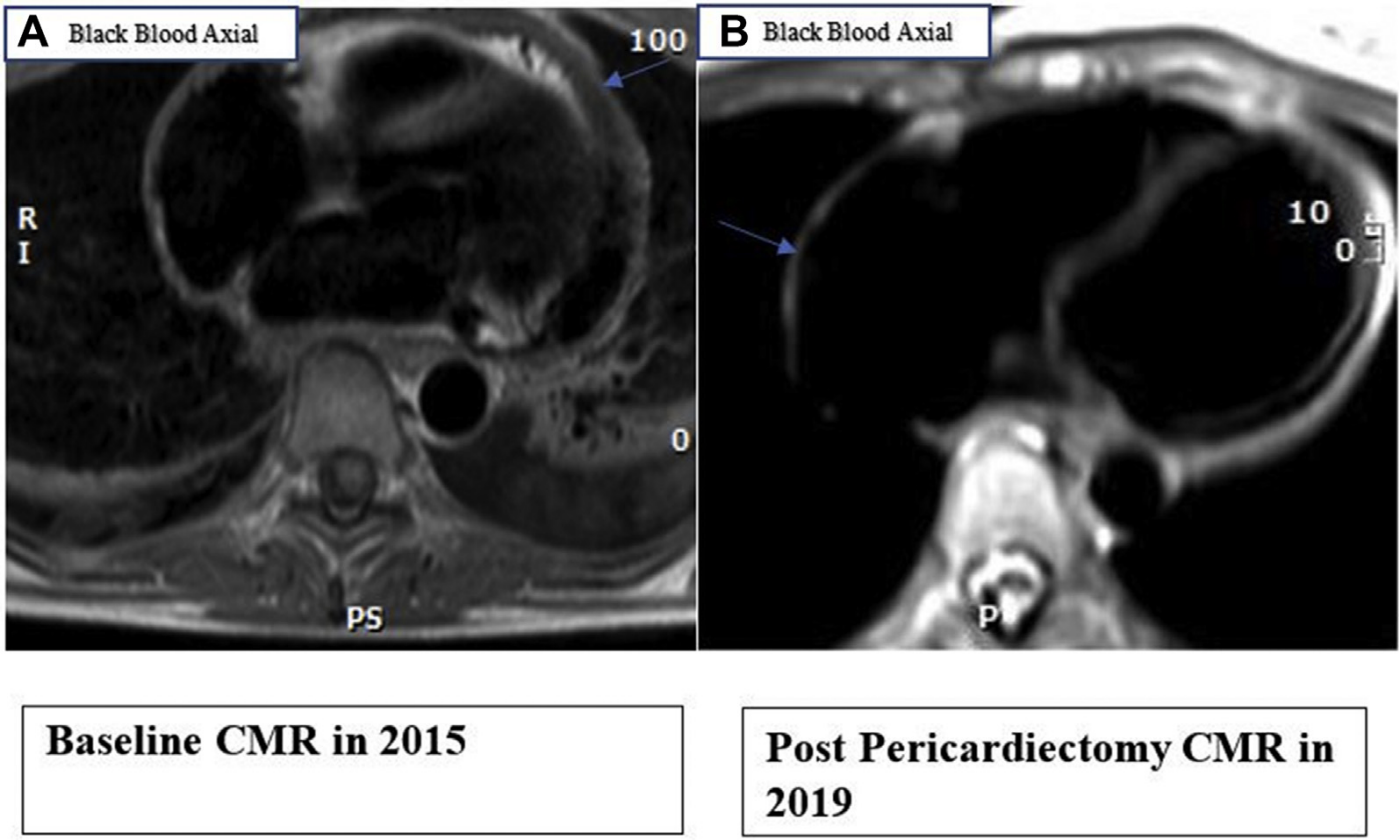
Cardiac MRI Black Blood Axial imaging (A) 2015 image showing increased pericardial thickening. (B) 2019 postpericardiectomy image; blue arrow demonstrates resection of pericardium.
